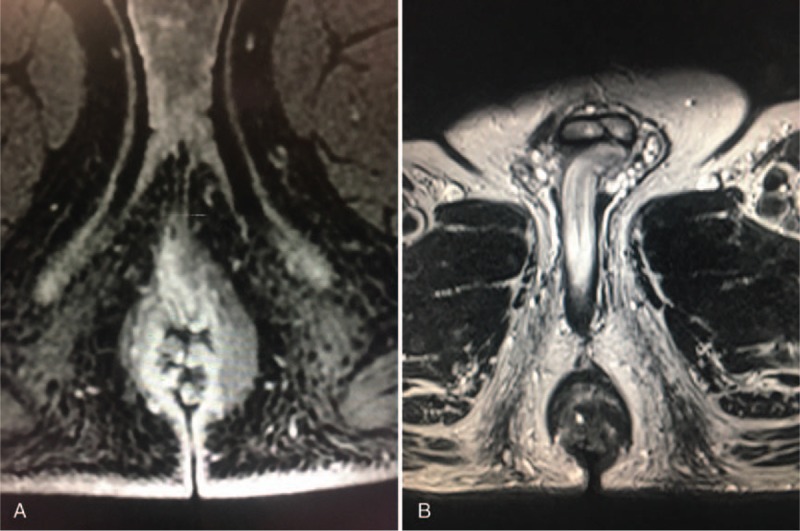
Pelvic MRI scan performed before (A) and after (B) supplementation with rectal and oral probiotics. MRI = magnetic resonance imaging.
